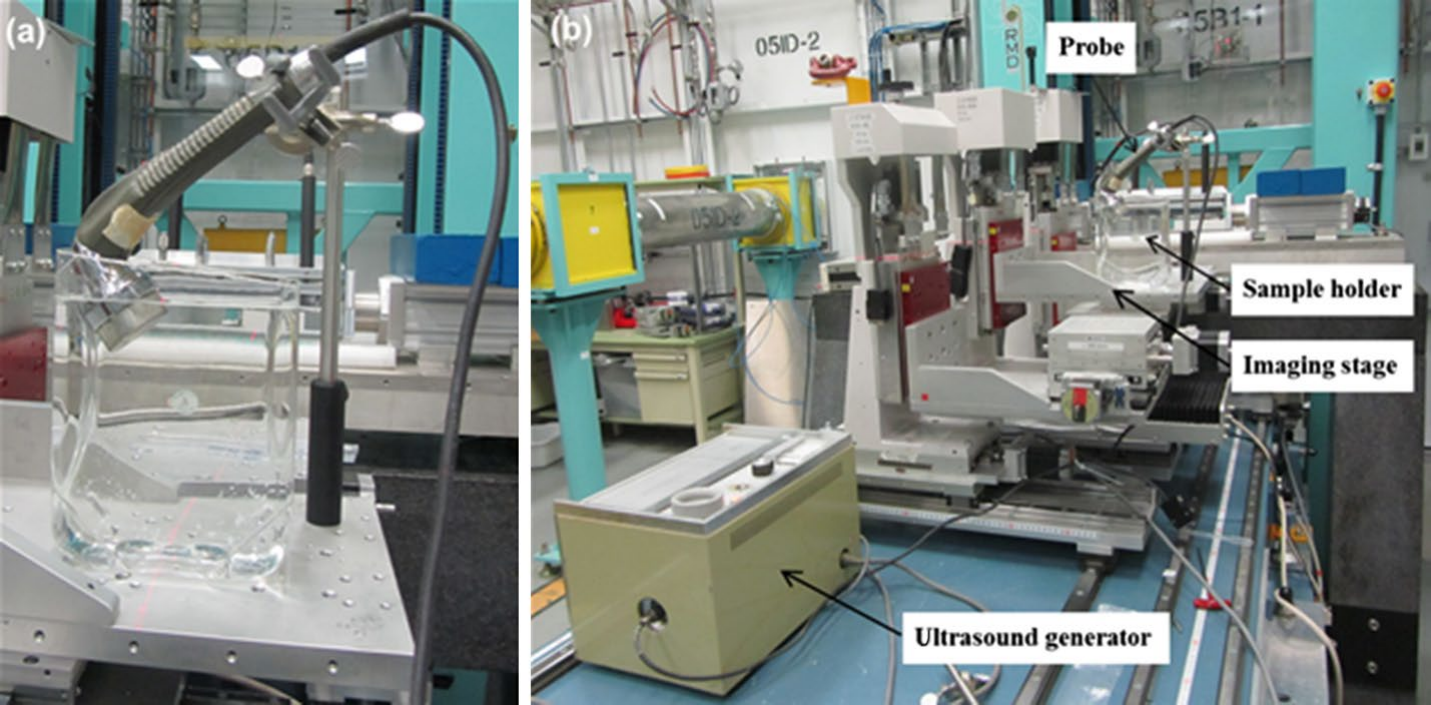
Preparation of the sample for therapeutic ultrasound treatment and X-ray imaging: a mounting the ultrasound probe on the scanning stage and inserting probe inside and at 45° angle of sample/water; b setting the signal generator/amplifier on the table and setting the experimental system
